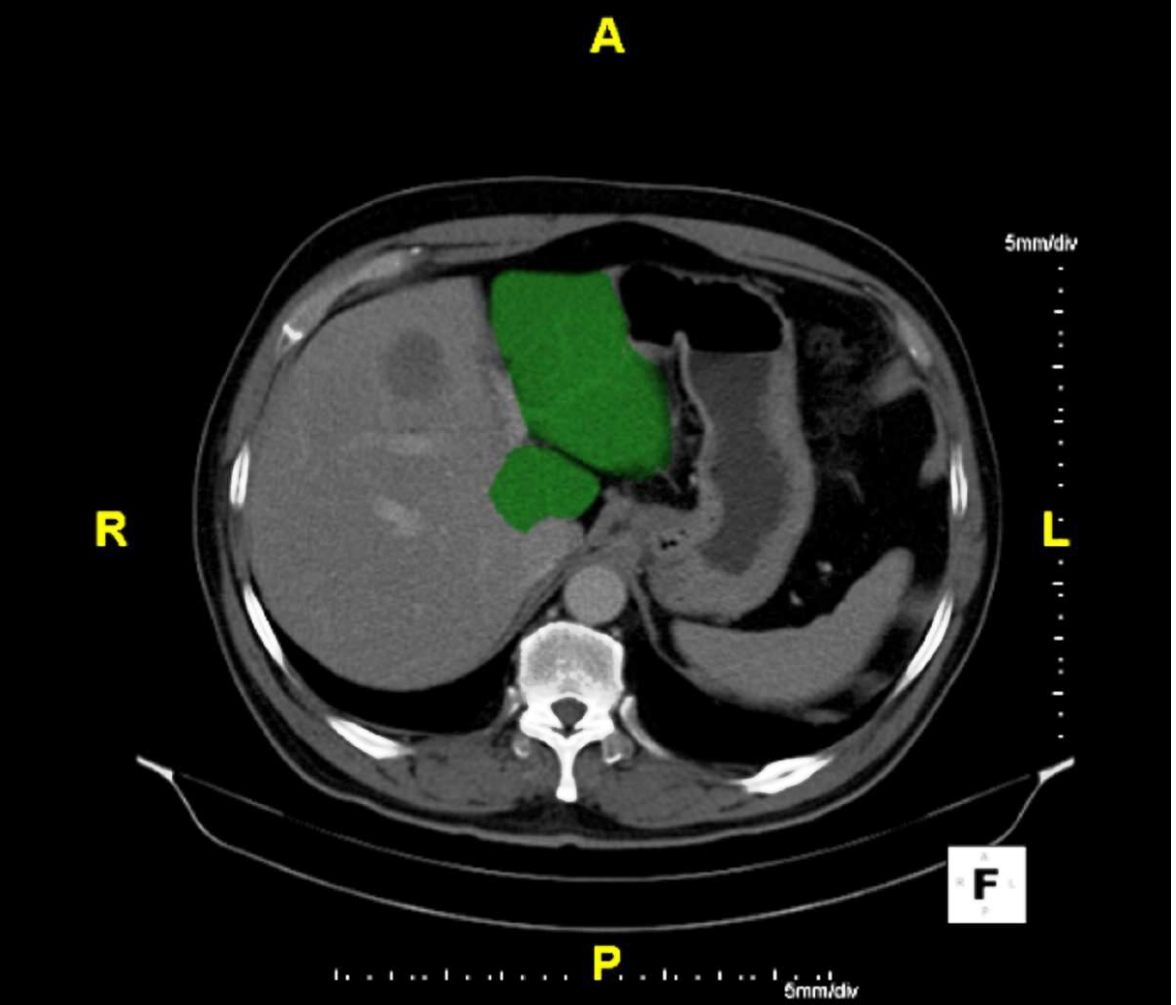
CLM with Small FLR Prior to Hepatic Resection. Patient with metastatic rectal carcinoma with right hepatic metastasis who was an operable candidate for right hepatectomy, but on preoperative computed tomography(CT; 5/12/09), the patient had a small left hepatic lobe and FLR.
